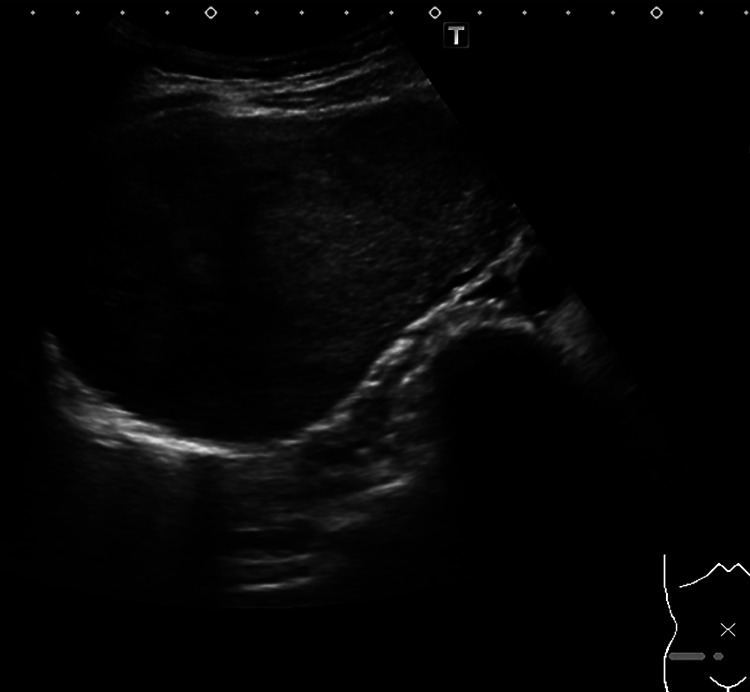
Abdominal ultrasound A multi-compartmented mass with 126×72-mm lining was observed in the right lower
